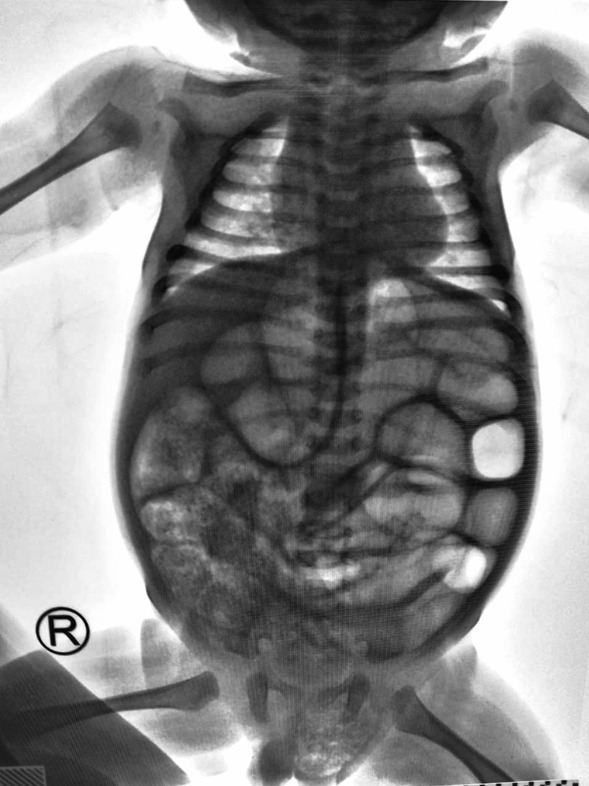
X-ray’s examination. This is the X-ray examination in the Emergency Department. We can see obstructed bowel and caecum in the left inguinal hernia
